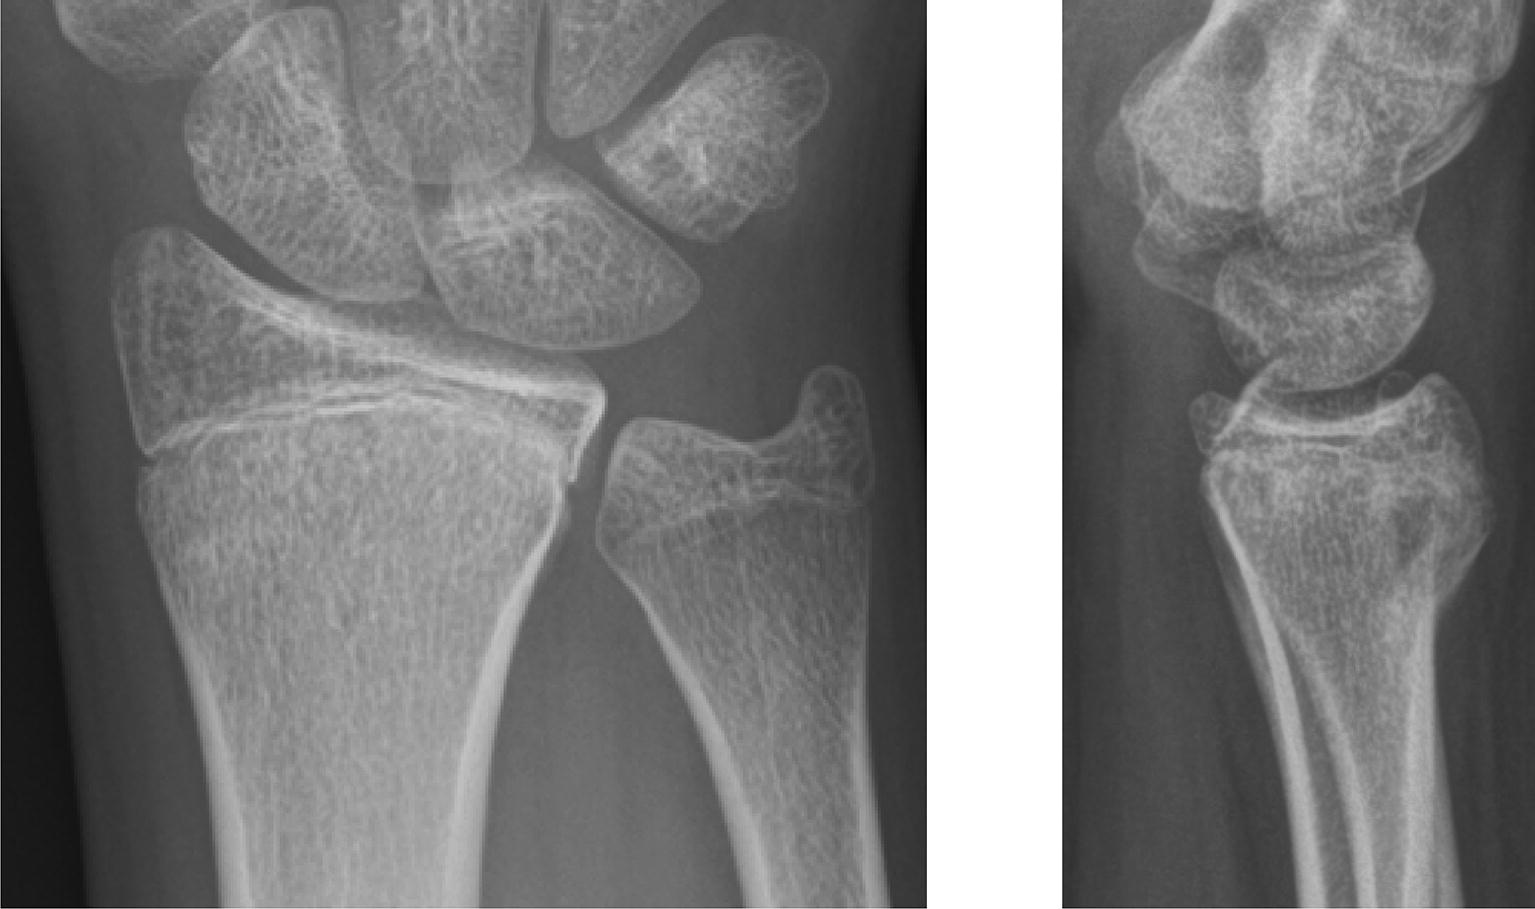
Follow-up imaging case 3. Radiograph showing healed fracture 3 months after trauma
